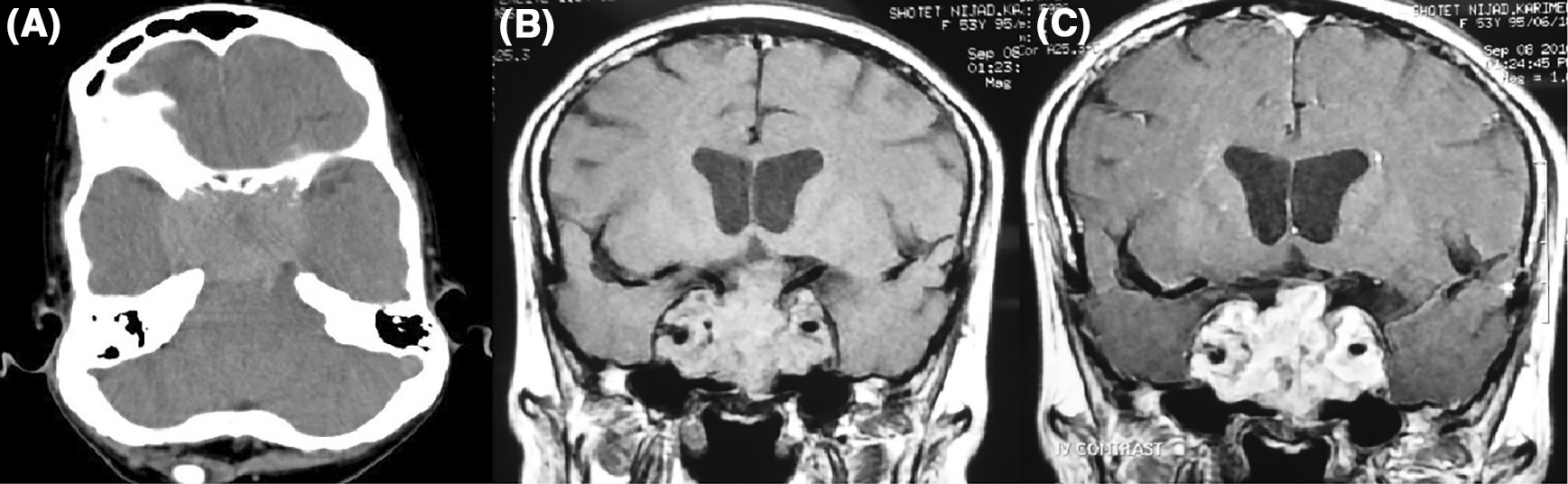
(A) Brain CT scan without contrast injection showing a hyperdense mass in the sellar/suprasellar region invading optic canals. (B) Coronal MRI views without gadolinium administration. (C) Coronal MRI with GD demonstrates a huge sellar tumor with suprasellar extension and bilateral cavernous sinus invasion.
